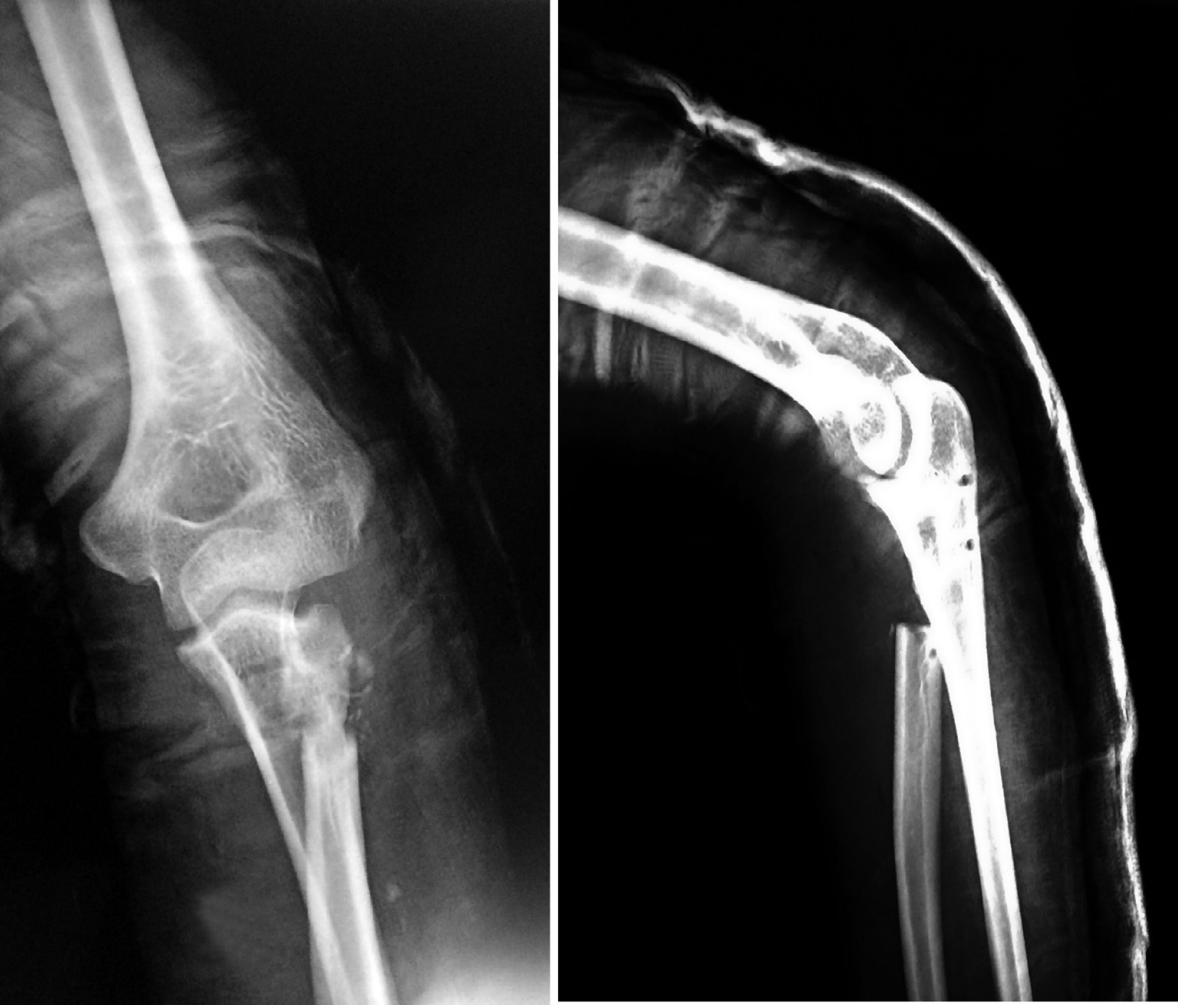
Postoperative radiograph after excision of radial head along with radial shaft up to the distal extent of synostosis. Drill holes seen near the osteotomized radius and ulna for securing the tensor fascia lata graft and muscles, respectively.
